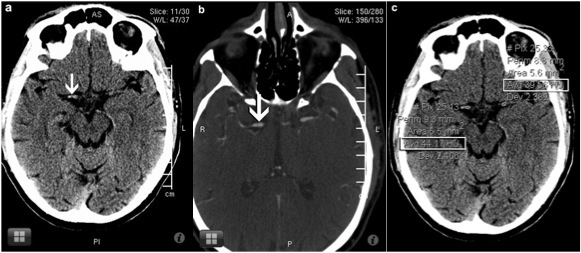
On an iOS device, an NCCT brain scan shown in image *a* was incorrectly interpreted as having a dense MCA sign (arrow). On an iOS device, a CT angiography brain scan (image *b*) demonstrates a normal patent vessel. On the workstation monitor, an NCCT brain image *c* shows nearly the same Hounsfield units (open rectangles) of both vessel segments, thus avoiding the error made on the iOS device.
